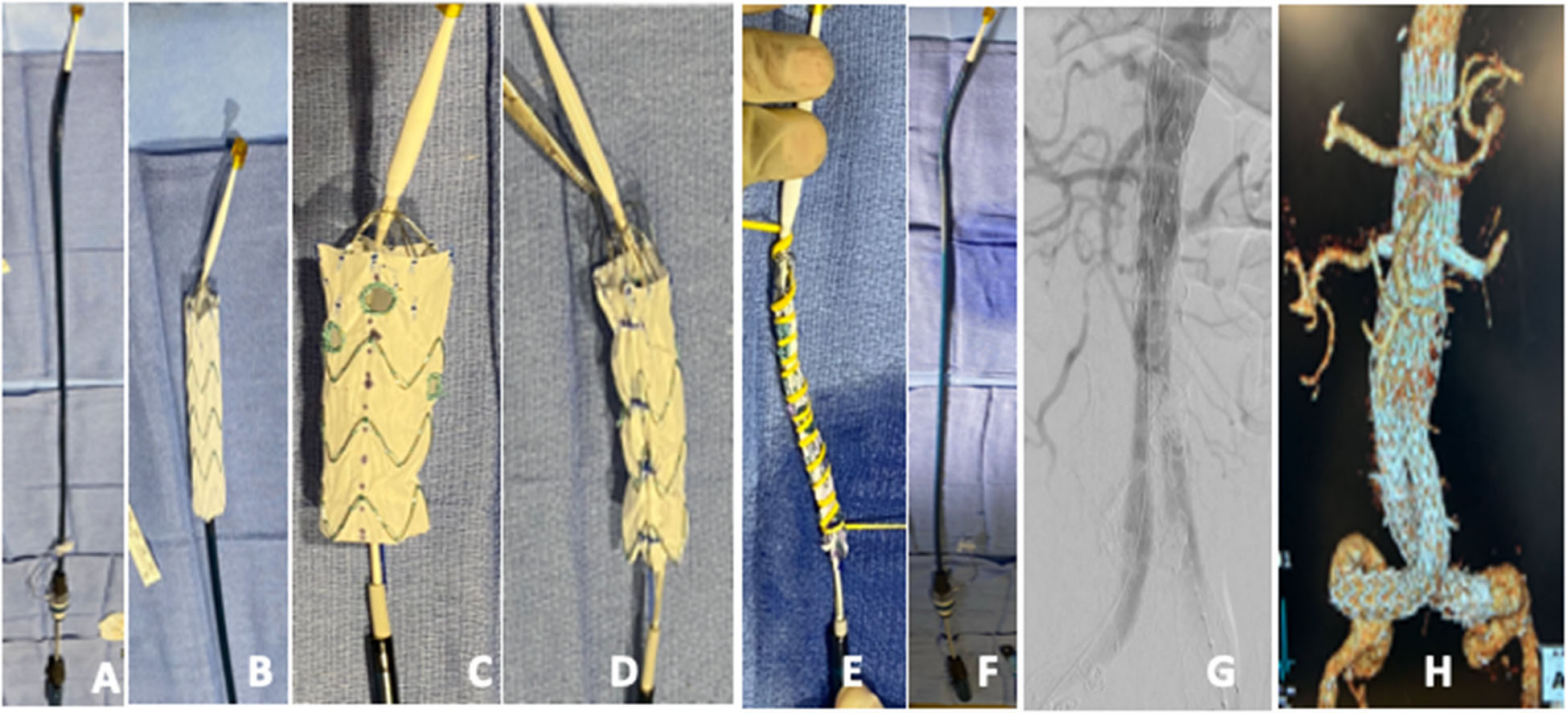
A-H. Steps of modification of the Zenith Alpha thoracic stent graft. The device is deployed on a sterile back Table (A). The bottom stent is cut with an ophthalmologic cautery to ensure adequate length to the flow divider of the failed stent graft. Fenestrations are created and reinforced as described in Fig. 1B (B). The device is posteriorly constrained at every Z stent (D), collapsed with a silastic loop (E), and resheathed (F). Completion angiography (G) and 3D post-operative CTA (H) show exclusion of the aneurysm, perfusion of target vessels, and no endoleak. Rationale for device selection: The existing stent graft was 24 mm in diameter, the visceral aorta measured 27 mm and the distance from the bottom of the celiac artery to the flow divider was 92 mm. Furthermore, iliac arteries were small and diseased. For this reason, the low profile Alpha thoracic stent graft ZTA-PT-30-26-108-W was the perfect fit for this 3 vessel repair after removal of the distal Z stent. Resheathing of this device requires removal of laser cut barbs, a process that is straightforward
